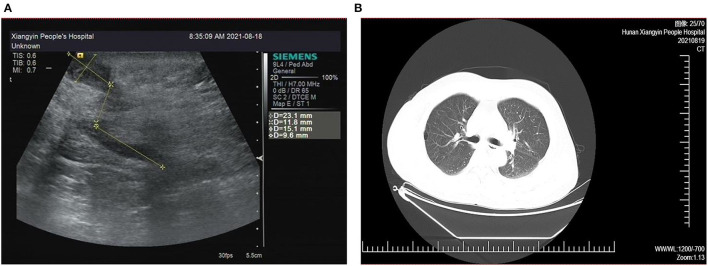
(A) Abdominal B-ultrasound results show the formation of sinus tract and empyema. (B) Chest computed tomography images of the lung window show no sign of tuberculosis-related diseases.
